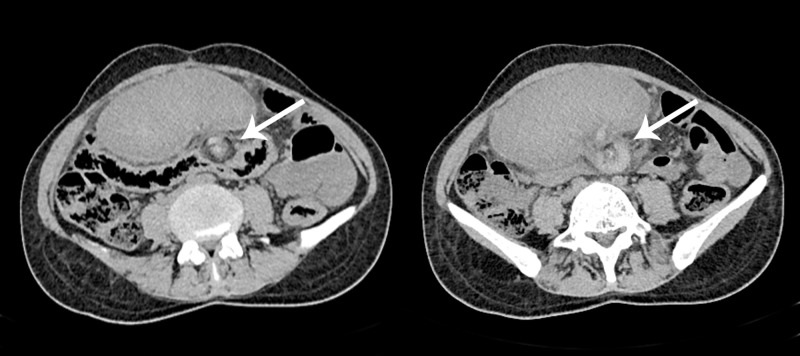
Non-contrast computed tomography images. Axial sections from the abdomen and pelvis showing hyperdense splenic pedicle and characteristic whorled appearance of the splenic vessels and fat, representative of torsion of wandering spleen (arrows).
